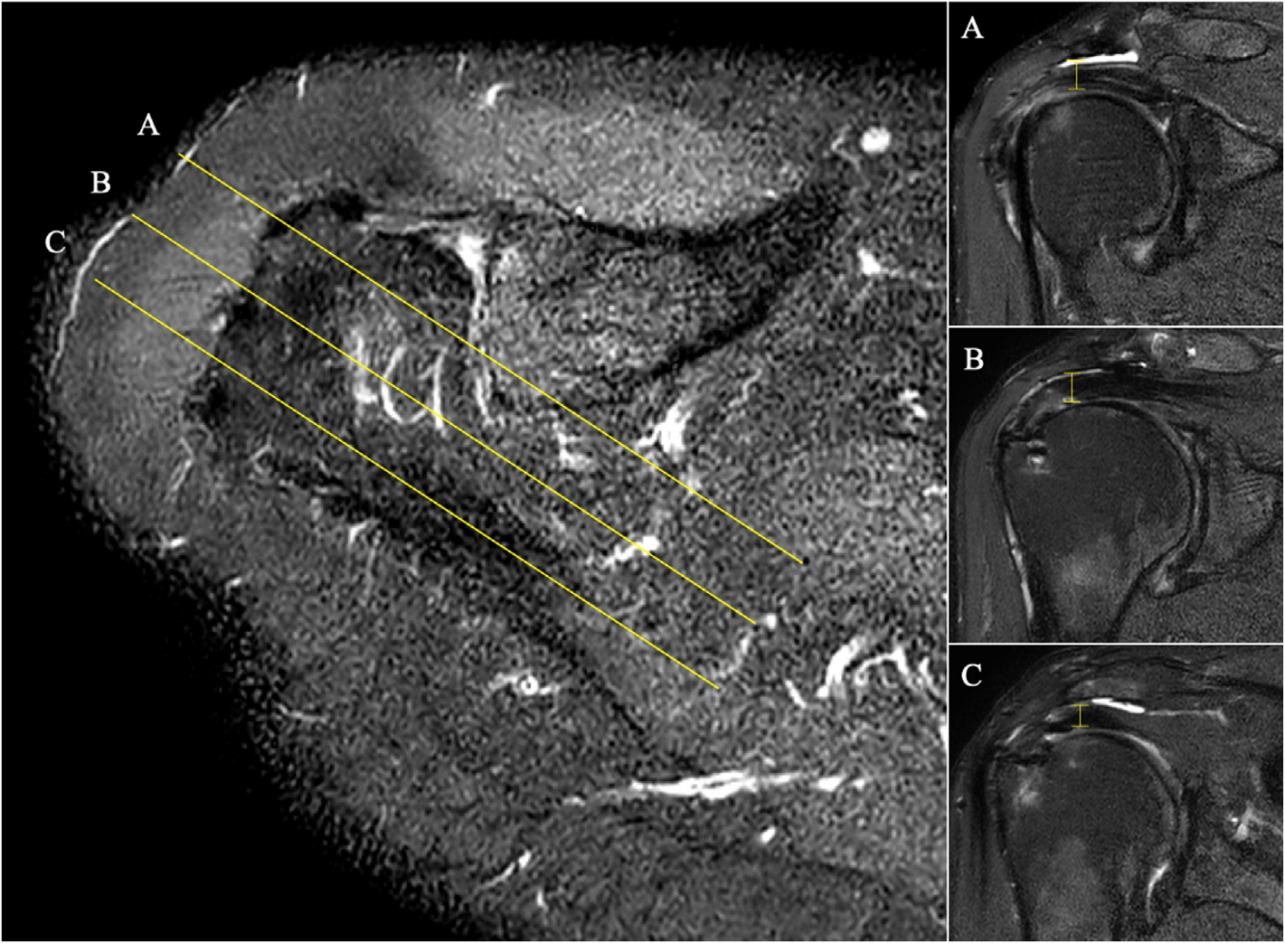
Axial view of the shoulder MRI showed regional AHD measurement which was performed in three ROI: anterior third (A), middle third (B), and posterior third (C) of lateral acromion border according to the scapular plane.
